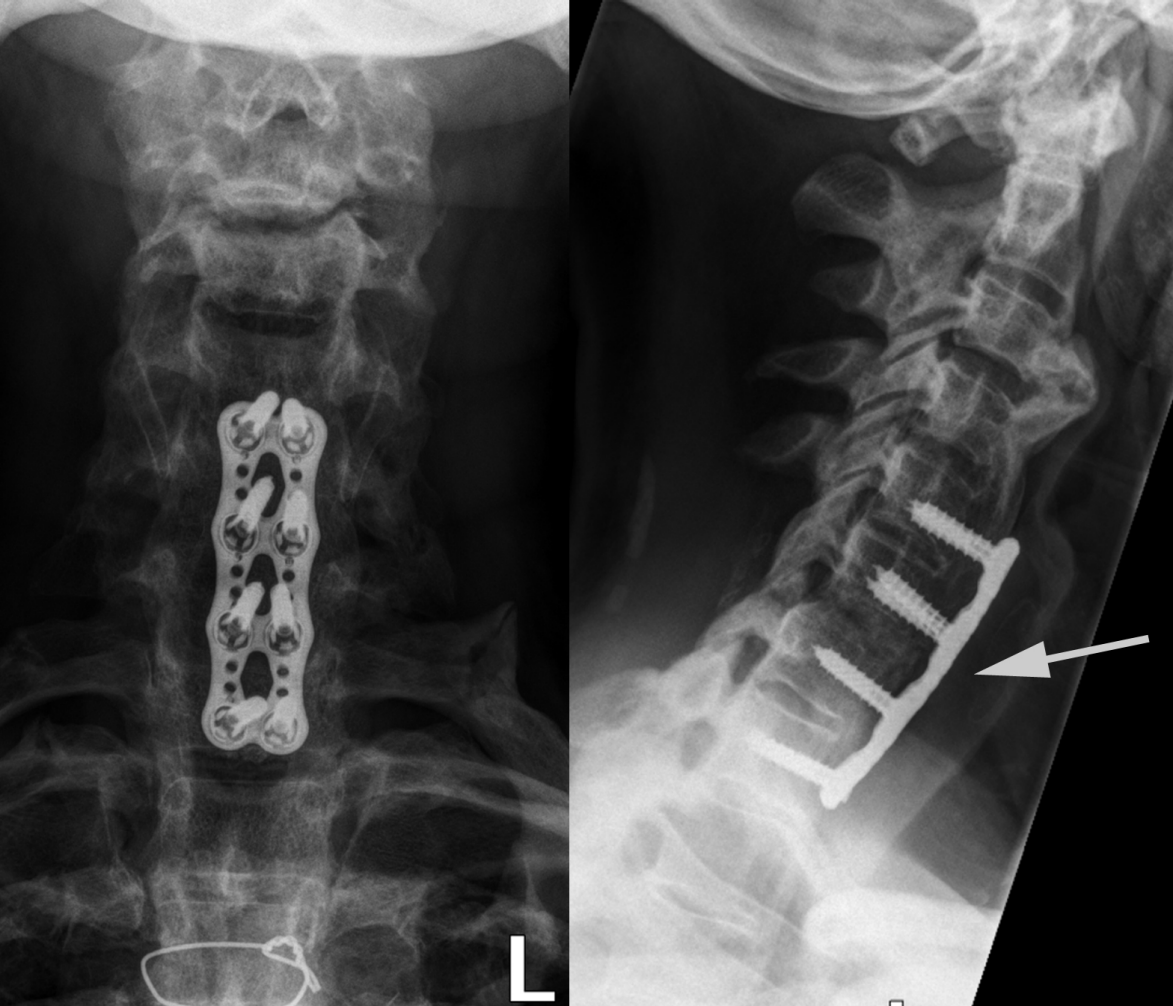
Plain radiographic images obtained at the six-month follow-up. The alignment is unchanged, the hardware intact, and there are signs of osseous consolidation.
